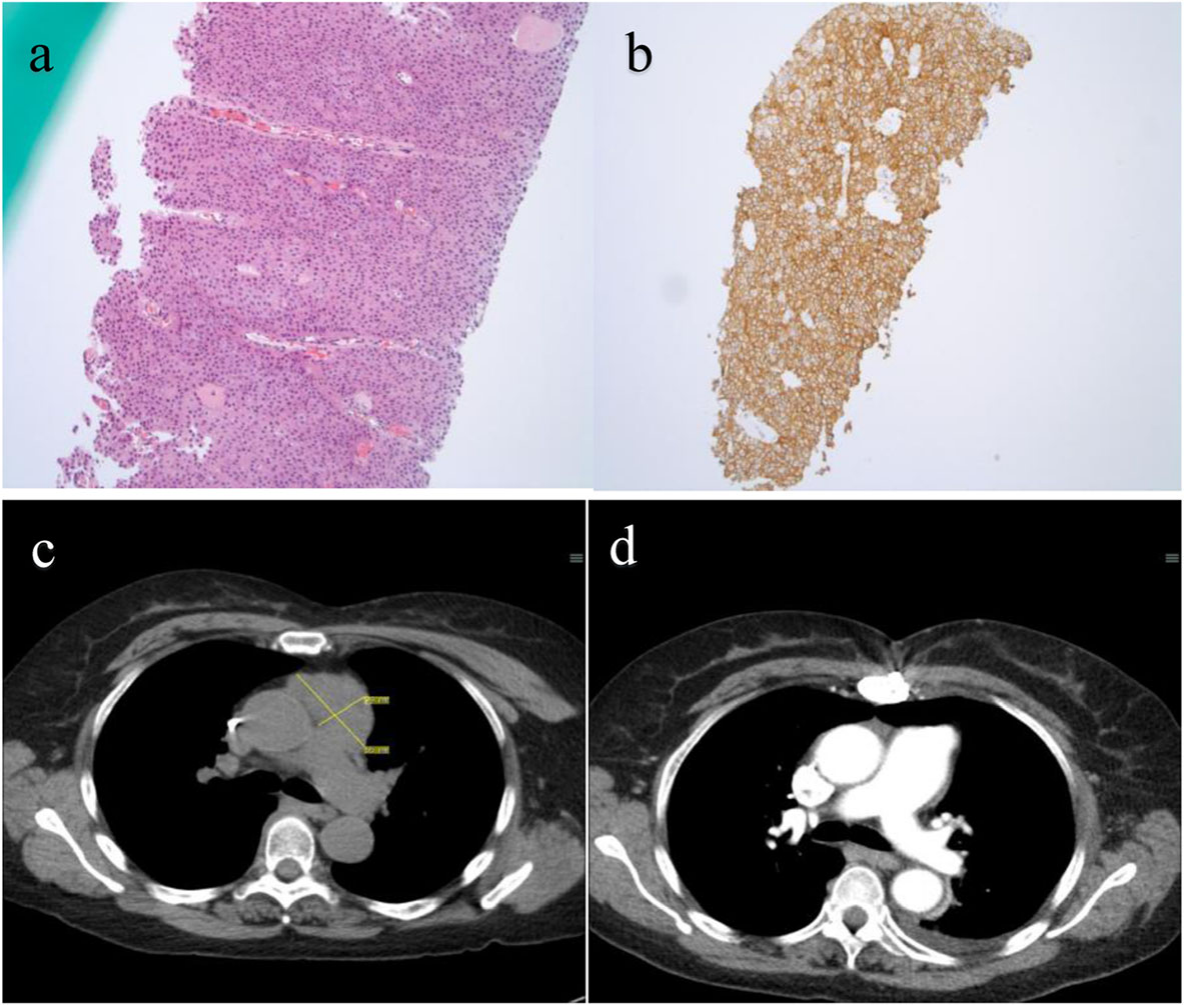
Pathology and Radiology findings or patient with low CD-4+ T-cell count (40 cells/ul). a. core biopsy of mediastinal mass suggestive of invasive squamous cell carcinoma. b. PD-L1 by IHC - 100% +. c. Pre-treatment CT chest suggestive of 5.5 × 2. 9 cm mediastinal mass. d. CT chest suggestive of CR with ICI therapy
